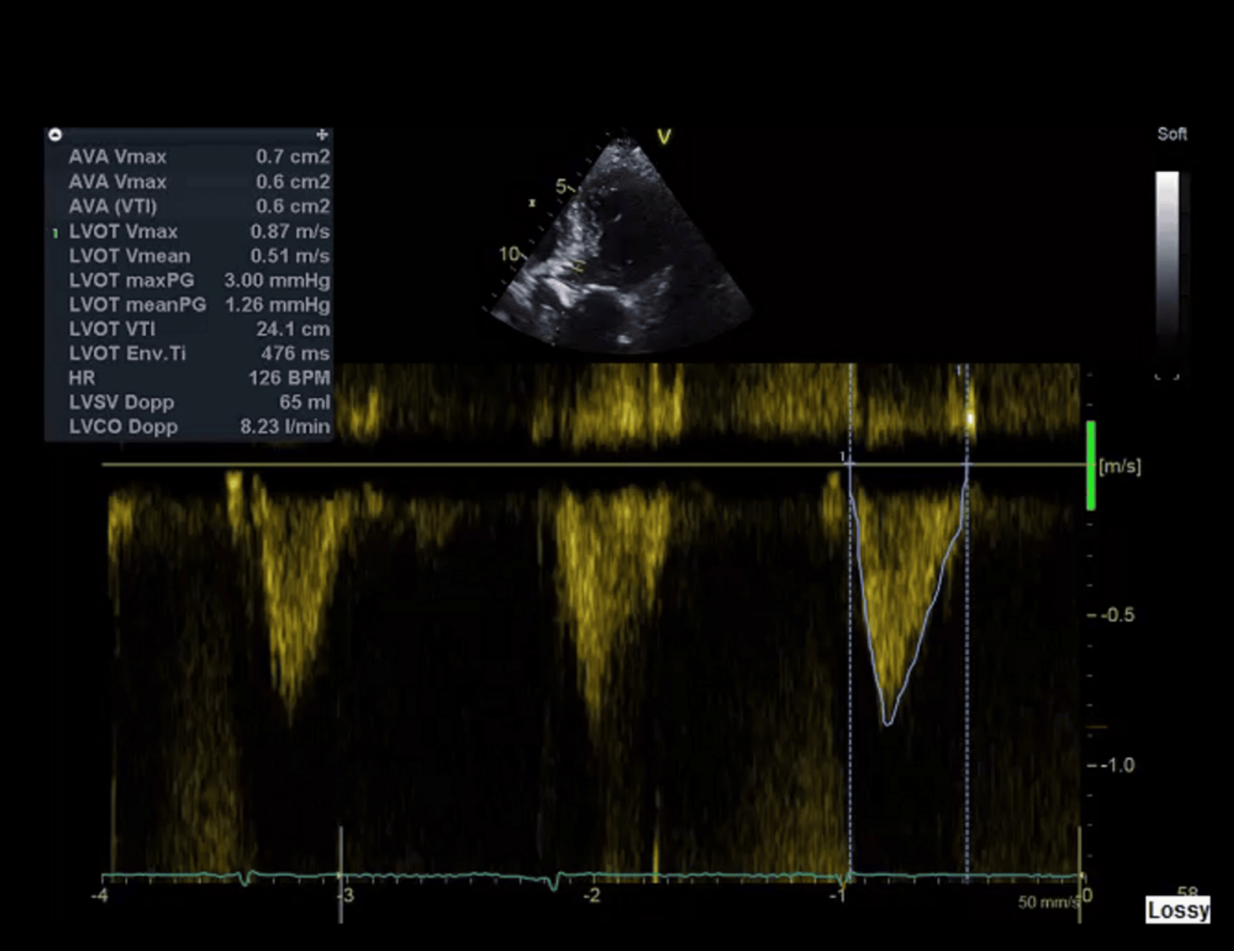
Echocardiograph showing indexed aortic valve area=0.6 cm².
